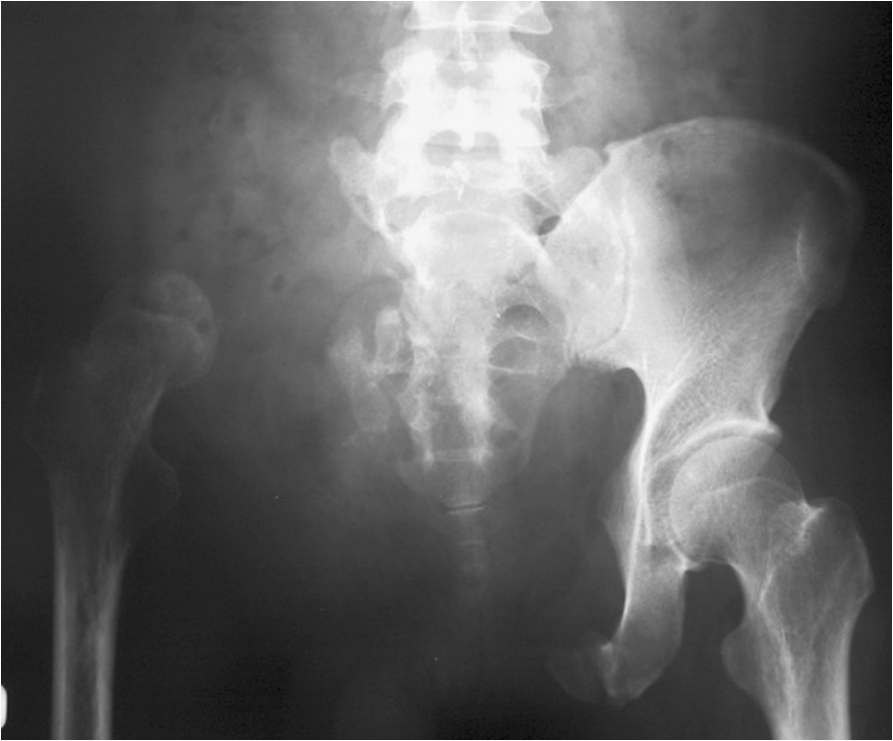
Anteroposterior radiograph of the pelvis postinternal hemipelvectomy showing an upward migration of the head of the right femur and tumor margin extending through the right sacral foramens.
